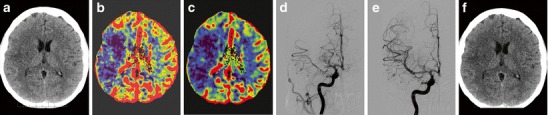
A 41-year-old obese, normotensive female treated with hormonal contraception developed left-sided hemiplegia at 6 a.m. a Acute CT at 7:30 a.m. shows a mild hyperdense lesion in the distribution of the right MCA and a large perfusion deficit with no penumbra on b CBF and c CBV maps. At 8:20 a.m. intravenous Actilyse was applied, and at 10:35 a.m., despite the absence of penumbra, d mechanical thrombolysis of the obliterated right MCA was performed e with successful thrombus removal. Left-sided hemiplegia resolved within a week. Six weeks after the stroke the patient was fully independent, going to work, driving a car and feeling no limitation of her left extremities except for “slight clumsiness” of her left hand. f CT scan with minimal residual ischemic changes. MCA, middle cerebral artery; CBF, cerebral blood flow; CBV, cerebral blood volume
